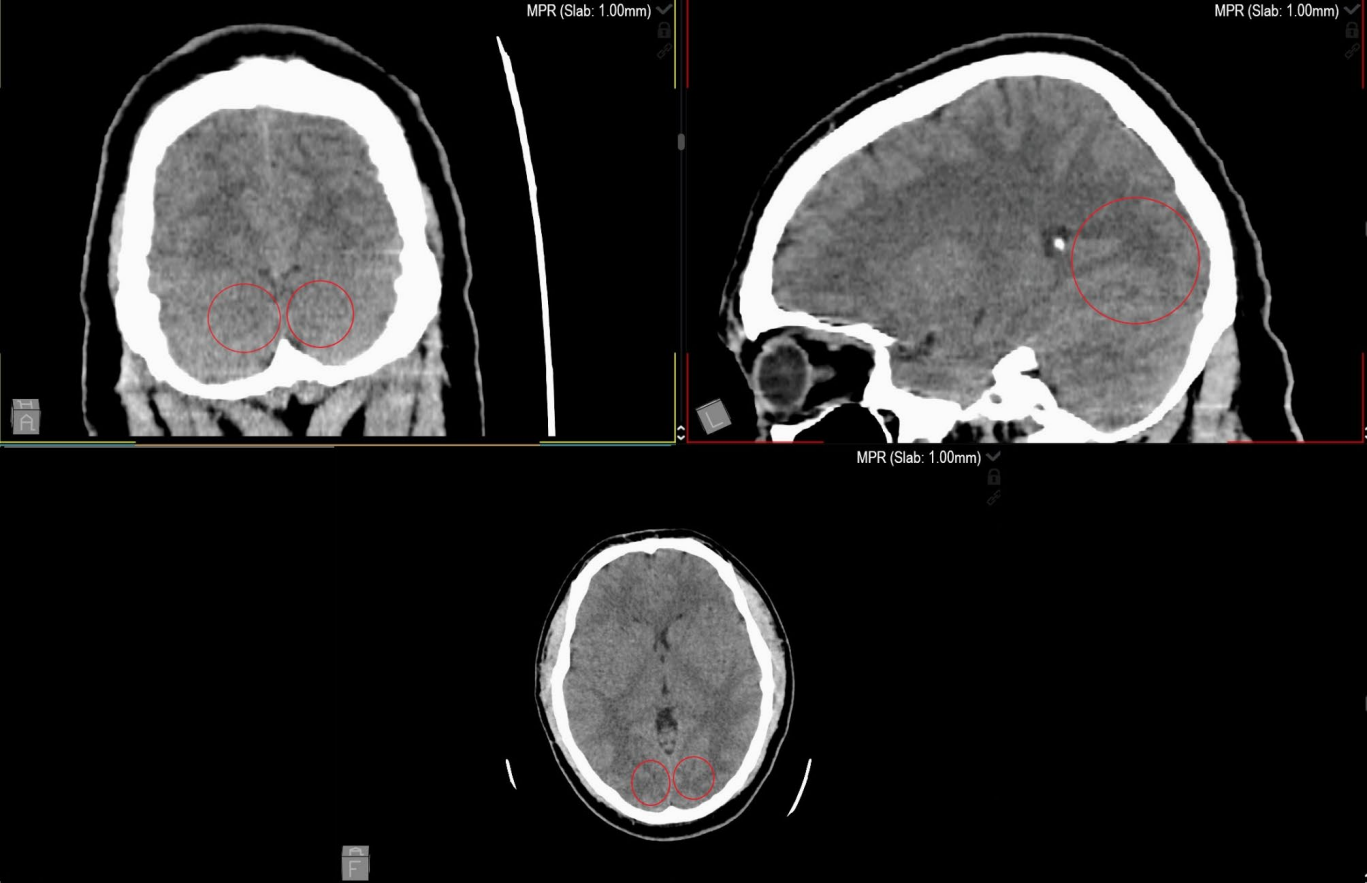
Male head CT with bilateral hypodensity in white matter, acute toxic leukoencephalopathy
